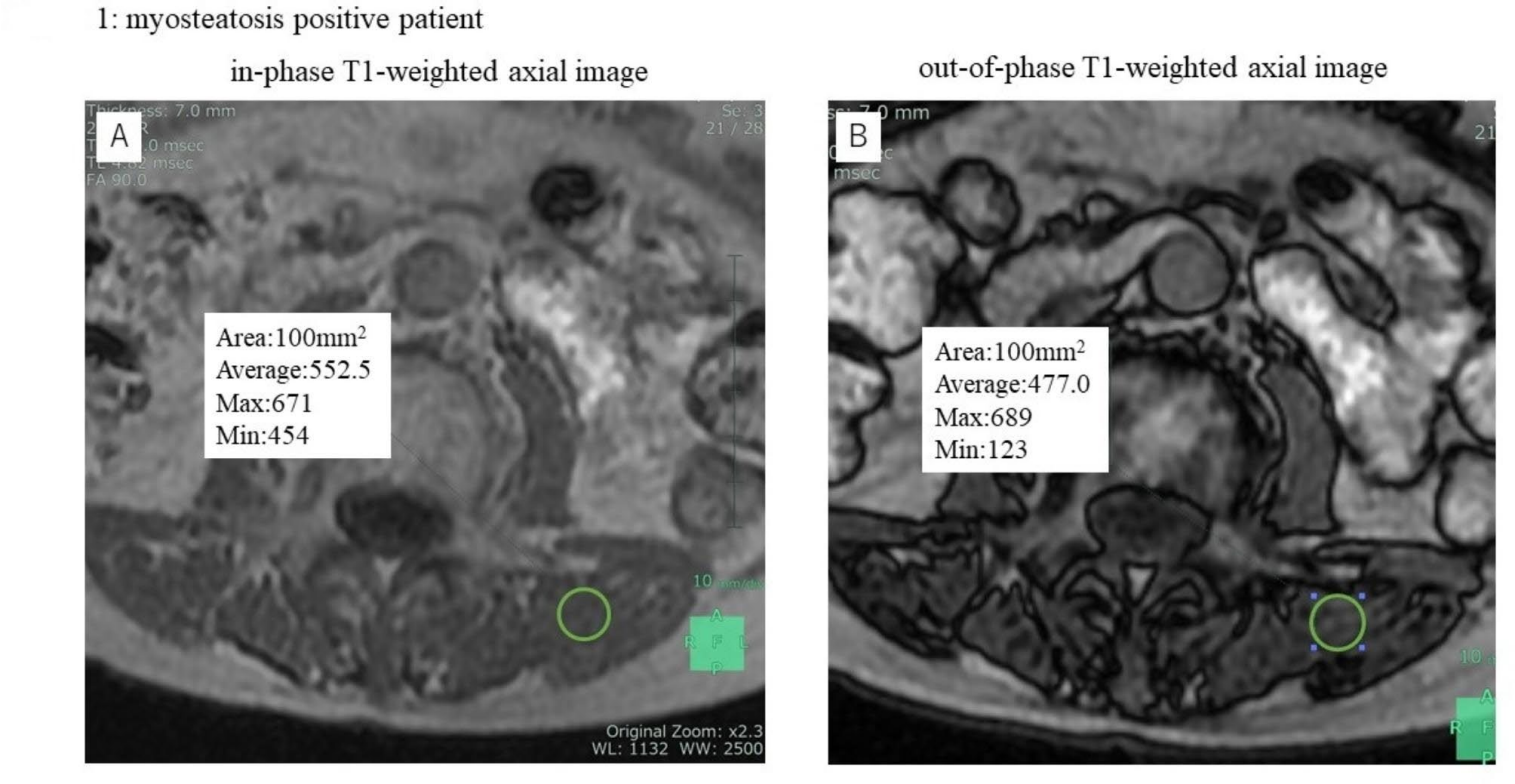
The representative MRI findings in myosteatosis positive patient in-phase T1-weighted axial image out-of-phase T1-weighted axial image
